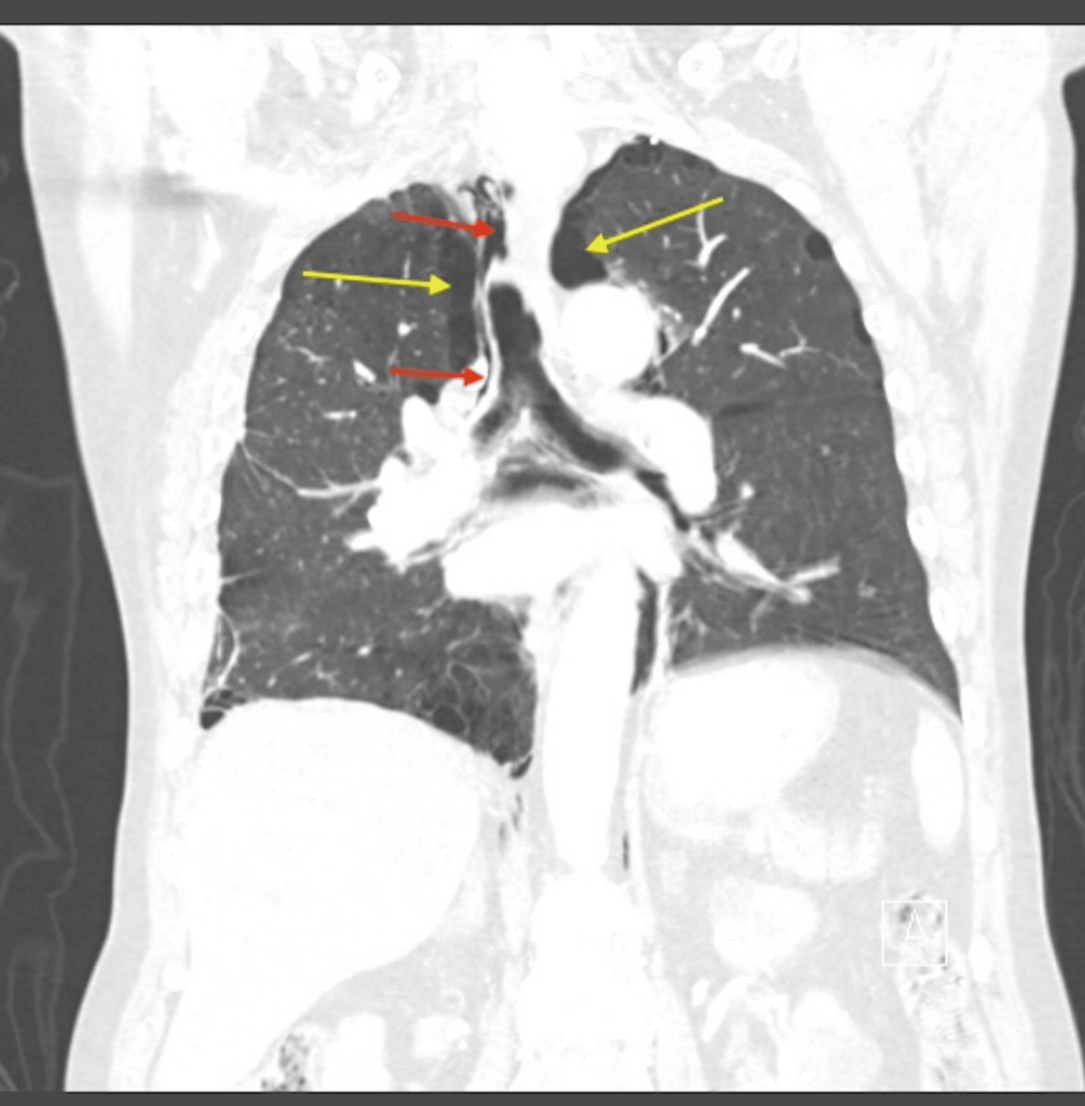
Coronal view of CT angiography showing pneumomediastinum and bilateral pneumothoraces Red arrows indicate pneumomediastinum, and yellow arrows indicate pneumothoraces CT: computed tomography
